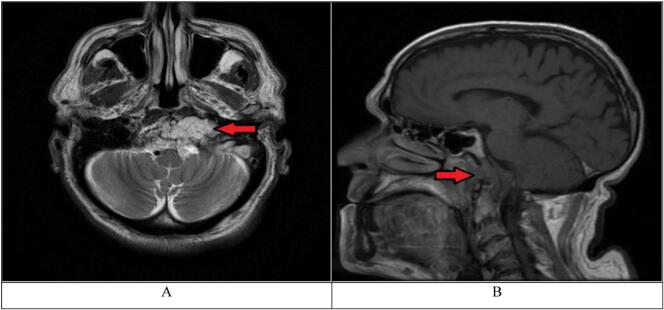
A. T2-weighted MRI, axial view. The arrow shows hyperintense Clival lesion located 3 mm from the anterior end of the clivus, the mass can be seen compressing the left internal carotid artery. (pre-operative MRI). B. T1-weighted MRI, sagittal view. The arrow shows hypointense mass causing severe destruction of the clival cortex, the mass can be seen compressing the anterior aspect of the medulla. (pre-operative MRI).
